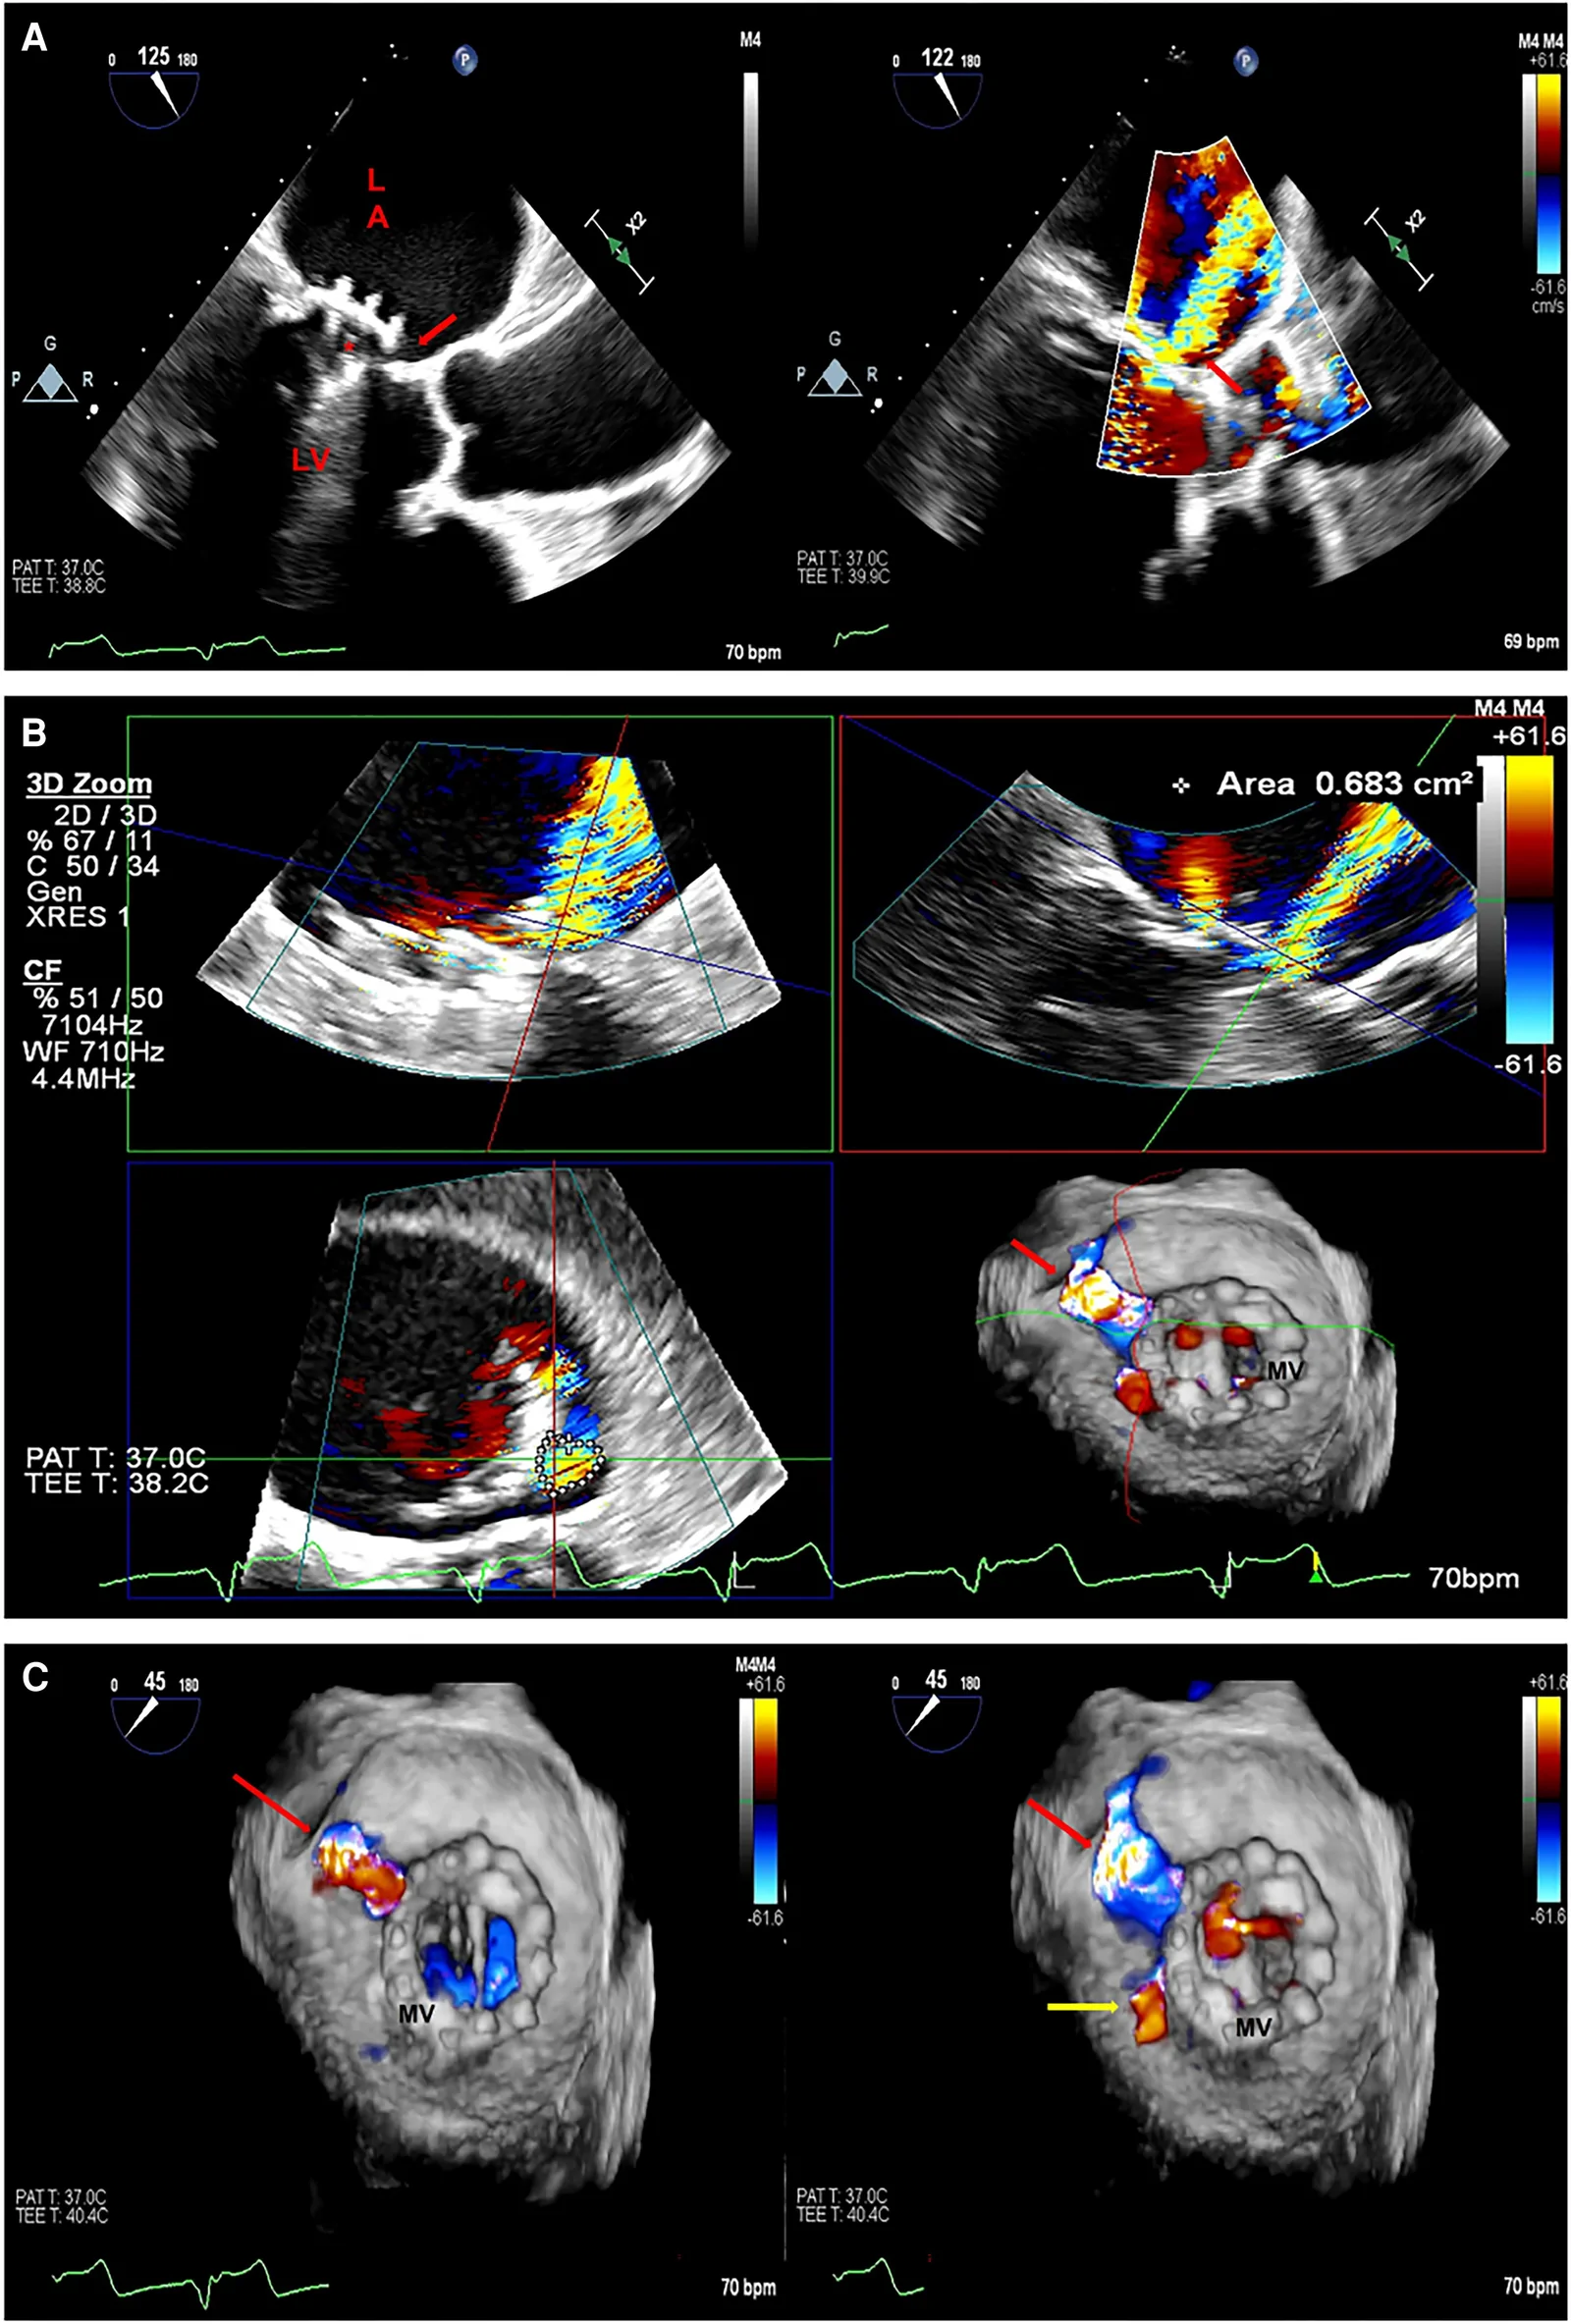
2D TEE long-axis view with and without colour Doppler shows a mechanical mitral prosthesis (asterisk) with anterolateral paravalvular leak (red arrow) (*A*). Three-dimensional multiplanar reconstruction confirms the anterolateral periprosthetic regurgitation (red arrow) (*B*). Real-time 3D-TEE colour Doppler from the atrial perspective demonstrates the anterolateral regurgitation adjacent to the prosthesis at eleven o’clock (red arrow) and a smaller posterolateral defect at nine o’clock (yellow arrow) (*C*).^1^ LA, left atrium; LV, left ventricle; MV, mitral valve.^35^
